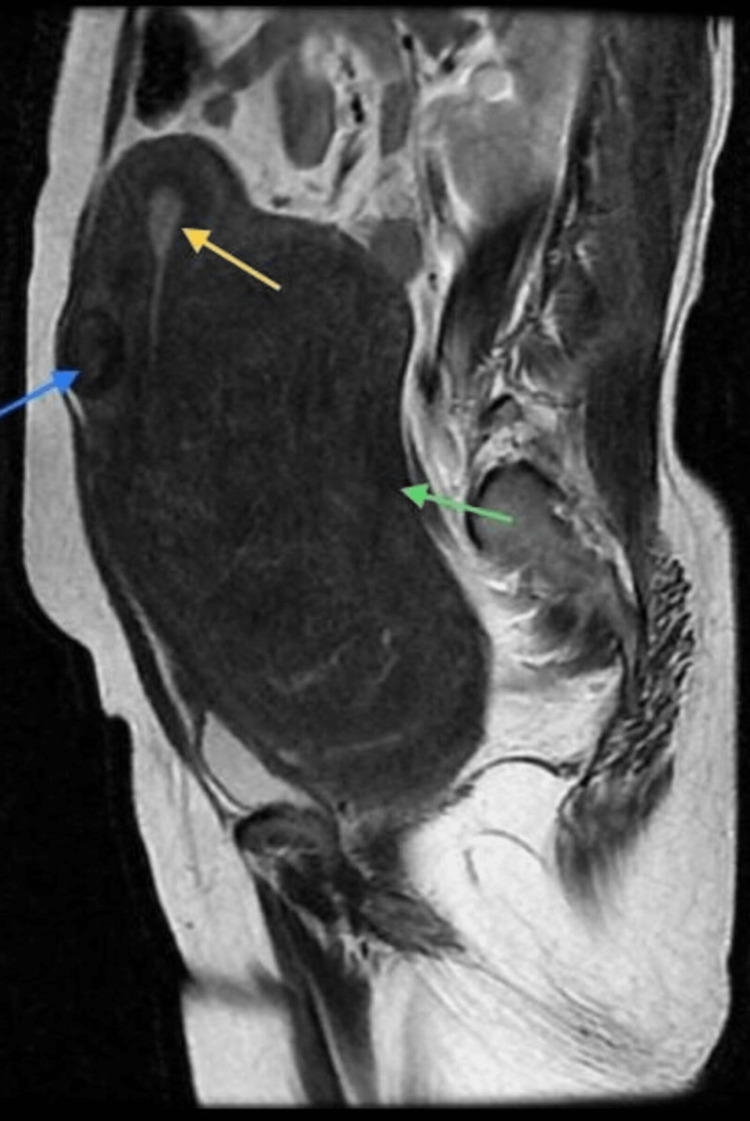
A large posterior wall uterine fibroid (green arrow) displacing endometrium anteriorly (yellow arrow) and a small anterior wall fibroid (blue arrow) on T2-weighted MRI.
